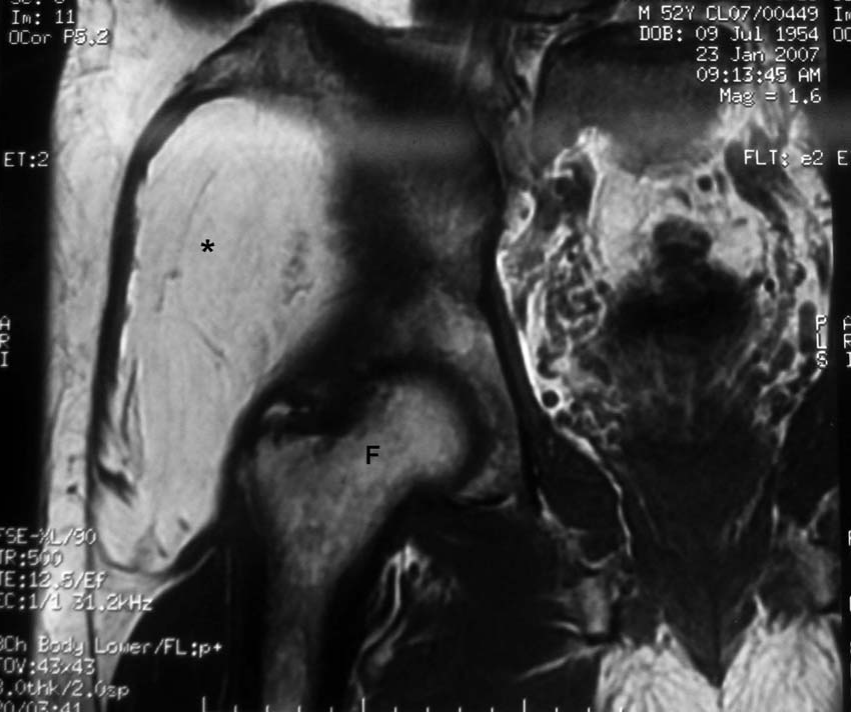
Magnetic resonance imaging (coronal view) showing the lipoma and the head of the right femur.
